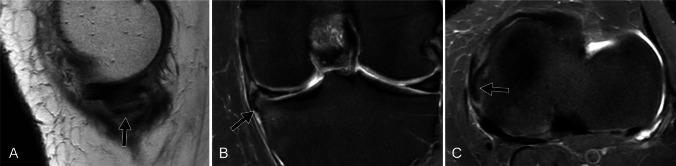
Horizontal flap tear in a 58-year-old man. Sagittal proton-density-weighted MRI (A), coronal proton-density-weighted fat-suppressed MRI (B), and axial proton-density-weighted fat-suppressed MRI (C) demonstrate horizontal flap tear of the body of the medial meniscus with meniscal flap (arrows) displaced into the medial meniscotibial recess. Note the bone marrow edema-like signal along the medial margin of the tibia underlying the flap, which can be a useful secondary sign when looking for a tear
